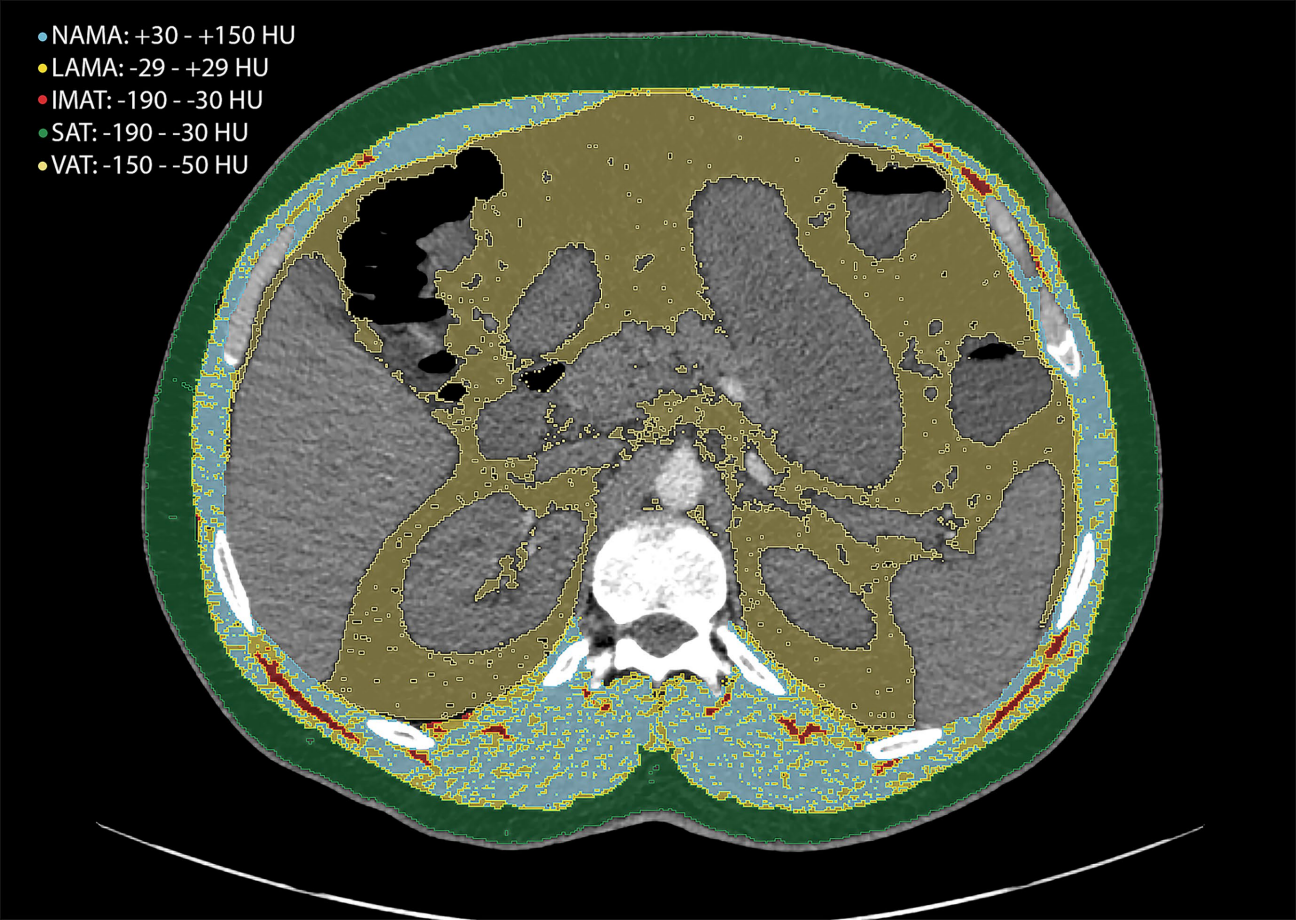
Computed tomography (CT) images at the level of the 12th thoracic vertebra were used for the quantification of intermuscular adipose tissue (IMAT), normal attenuation muscle area (NAMA), low attenuation muscle area (LAMA), subcutaneous adipose tissue (SAT), and visceral adipose tissue (VAT), expressed in Hounsfield units (HU).
